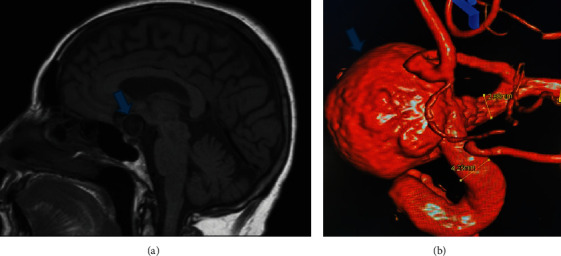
(a) MRI head without contrast showing a giant aneurysm (arrow) near the optic chiasm. (b) 3D CT angiogram confirming the presence of a giant aneurysm measuring 16 mm diameter with an 8 mm neck (arrow) arising from the internal carotid artery.
